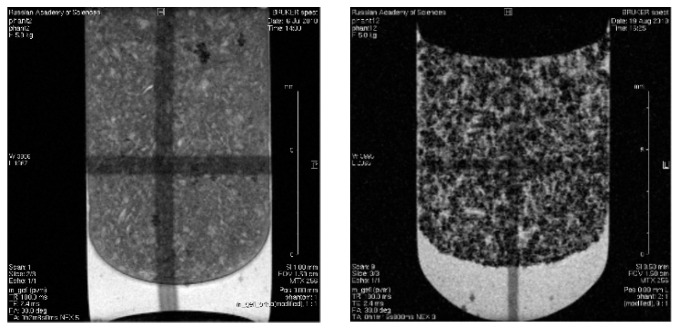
Gradient echo scans in MRI study of agar-agar gel (2% weight) and gel loaded by SPIONs@IFNα-2b. Dark points are the sites of accumulation of aggregated MPs.
